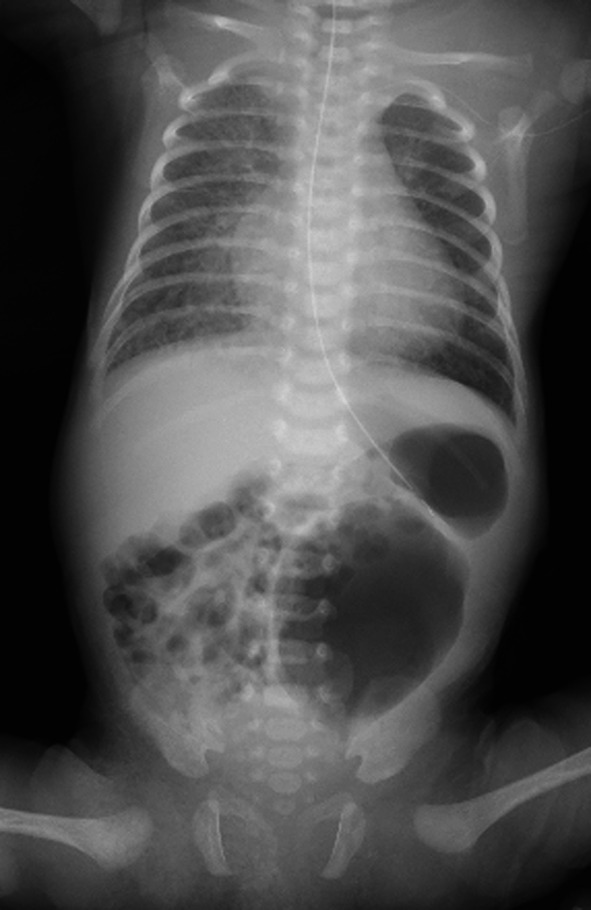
Abdominal X-ray 1 day after birth. The abdominal X-ray showed a dilated intestine in the left lower abdomen
